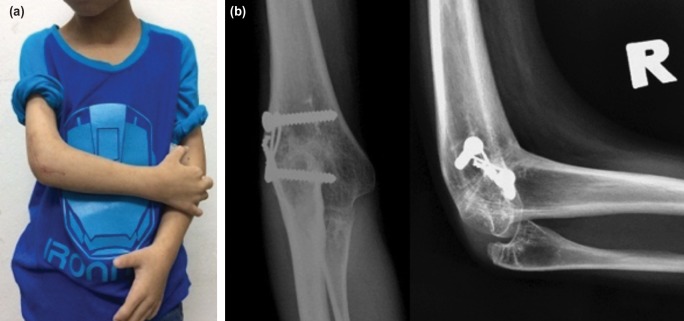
(a) Post-operative photograph and (b) plain radiographs taken one year after surgery showing right elbow position of 90 degrees and union of osteotomy site.
